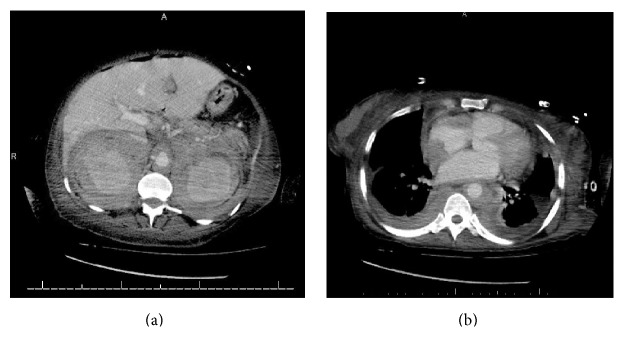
CT of the abdomen (a) showing extensive retroperitoneal soft tissue (fibrosis) surrounding the kidney and vessels. The soft tissue is seen extending into the mediastinum with involvement of the pleura, heart, and vessels (b).
